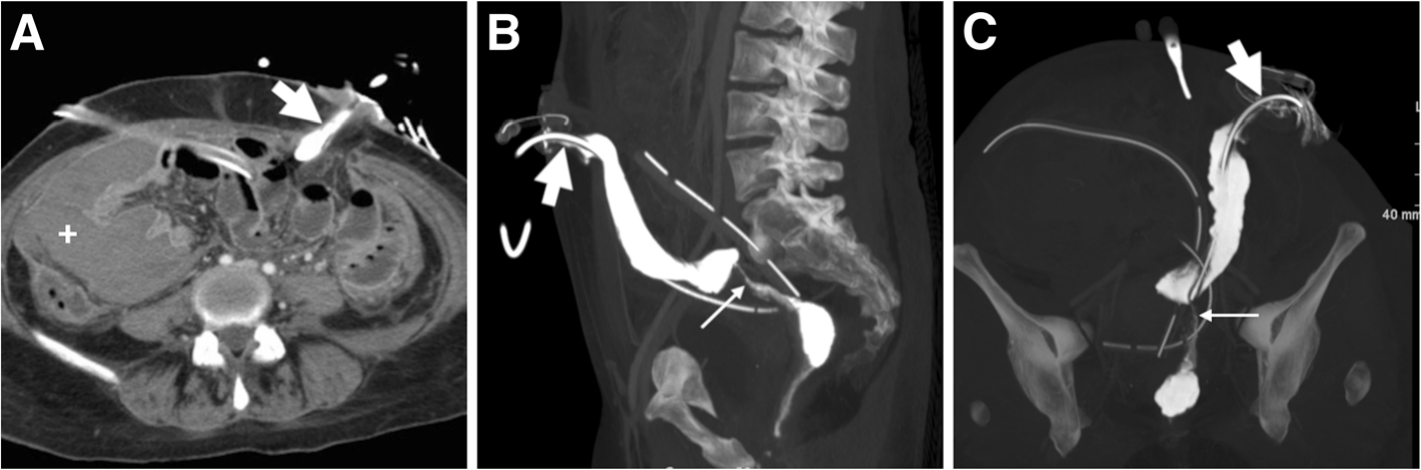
Combined CT plus WSC-SE in a patient with iatrogenic rectal perforation during stricture dilatation. Axial CT image (a) showing large hyperattenuating collection in the right abdomen (+) and diluted iodinated contrast medium injected in the cannulated efferent limb of the loop colostomy (thick arrows). Maximum-intensity projection reconstructions (b, c) depict the contrast medium-filled diverted rectosigmoid colon with stricture (thin arrows), without extraluminal leakage. Note drainage tubes in place
